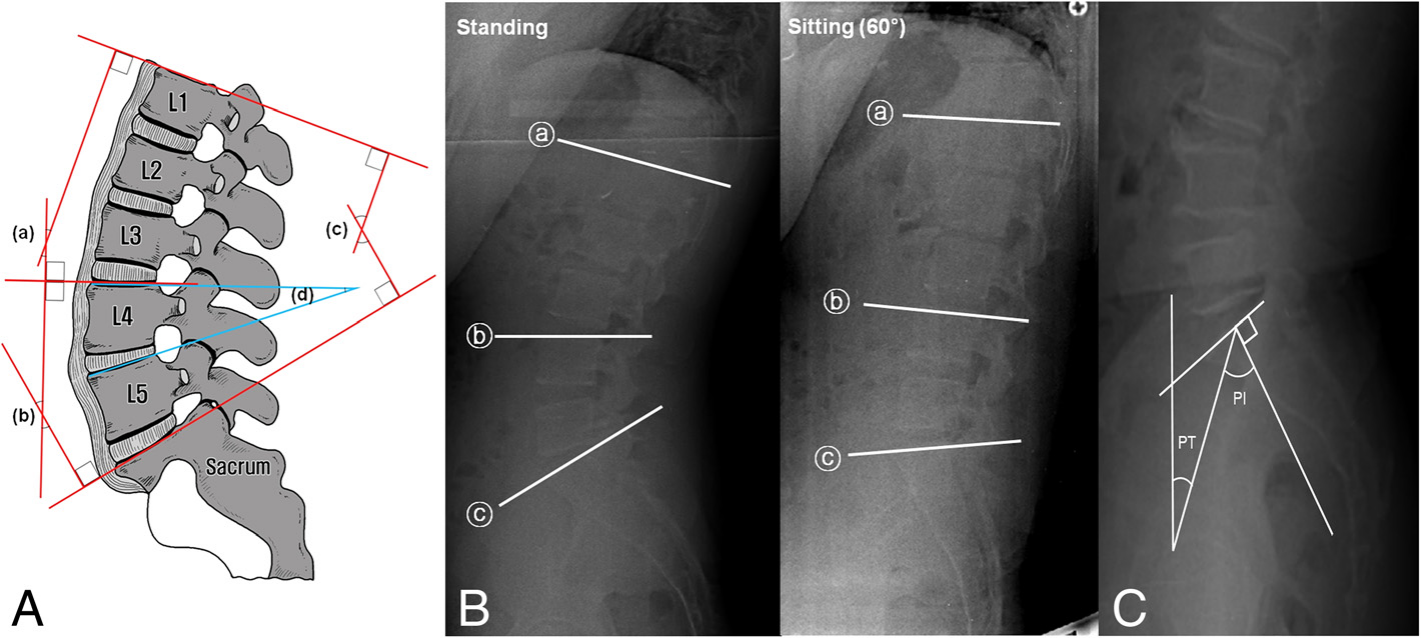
Lumbar lordosis and segmental angle measurements, radiographies on end-plate angle measurements and pelvic tilt and pelvic incidence measurements. (A) Measurement of lumbar lordosis, upper and lower lumbar lordosis, and segmental angles. (c) The lumbar lordotic angle is the angle between the upper plate of the first lumbar and first sacral vertebral bodies. (a) The upper lumbar lordosis is the angle between the upper plate of the first and fourth lumbar vertebral bodies. (b) The lower lumbar lordosis is the angle between the upper plate of the fourth lumbar and first sacral vertebral bodies. Each segmental angle was measured as the angle between the upper plates of two adjacent vertebral bodies. (B) Measurement references for end-plate angle based on radiography. In the standing position, lumbar lordosis (angle between ⓐ and ⓒ), upper lumbar lordosis (angle between ⓐ and ⓑ), and lower lumbar lordosis (angle between ⓑ and ⓒ) were 59.09°, 17.46°, and 44.82°, respectively. After position was changed to sitting with the seat back at 60°, lumbar lordosis, upper lumbar lordosis, and lower lumbar lordosis changed to 8.26°, -5.15°, and 15.03°, respectively. (C) Radiography showing the measurement of pelvic tilt (PT) and pelvic incidence (PI). PT is defined as the angle between the line joining the hip axis and the midpoint of the S1 end plate and the reference vertical line. PI is defined as the angle between the line joining the hip axis and the midpoint of the S1 endplate and the line orthogonal to the S1 end plate.
